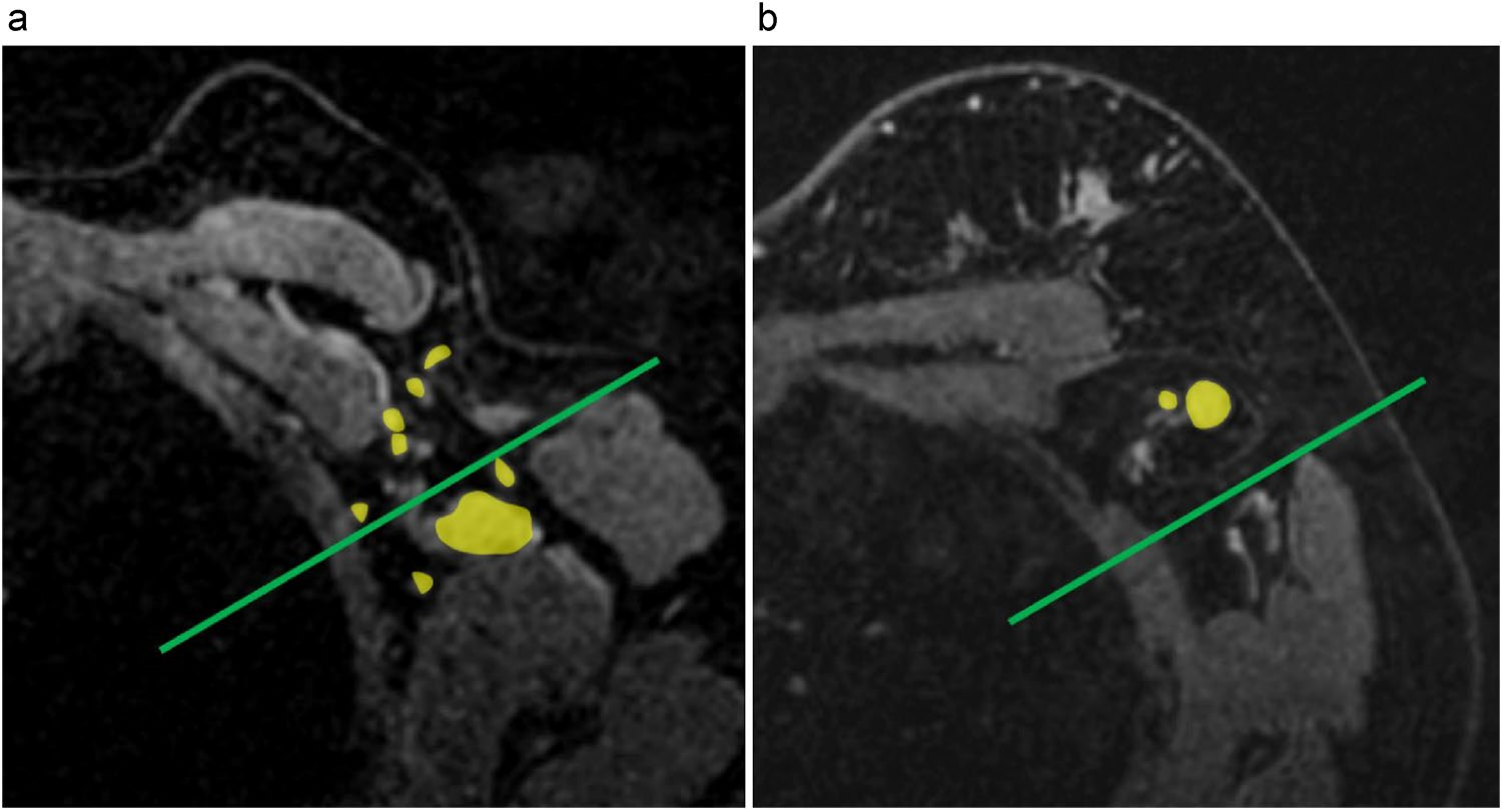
A representative MRI image of an axilla with post-vaccination lymphadenopathy and an axilla with lymph node metastasis. a Representative MRI image of the axilla with post-vaccination lymphadenopathy. b Representative MRI image of the axilla with lymph node metastasis. In the case of post-vaccination lymphadenopathy, significant lymphadenopathy was observed in the deep axilla. In the case of lymph node metastasis, lymphadenopathy was observed in the shallow axilla. *MRI* magnetic resonance imaging
